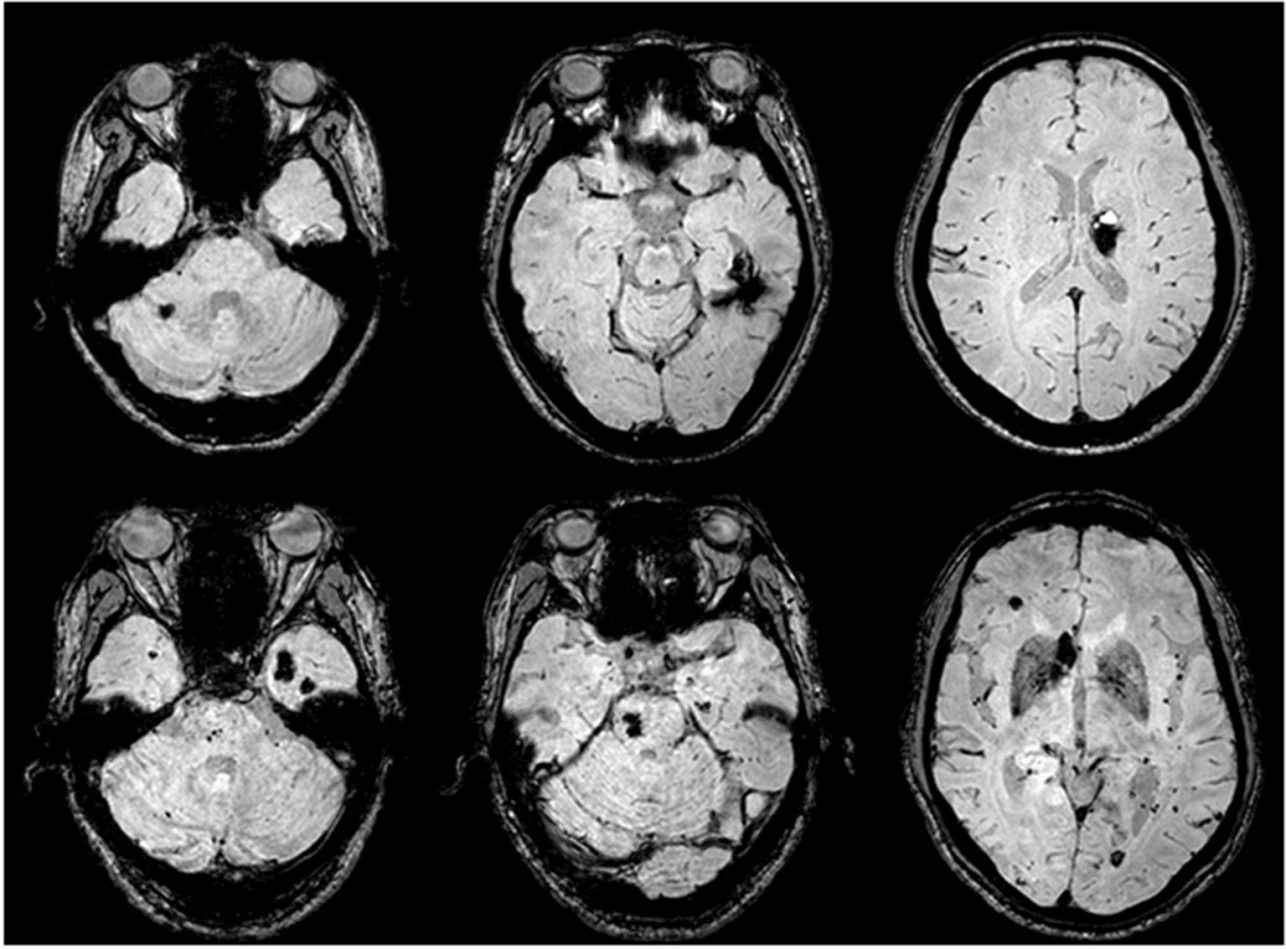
CCM lesions detected by SWI-MRI. The proband's SWIs (II:1) revealed dot and patchy low intensity lesions on temporal lobe, cerebellum, and thalamus (upper row). SWIs of the proband's father (I:1) demonstrated more widely-distributed multiple CCMs throughout the cerebrum, cerebellum, and pons (lower row).
